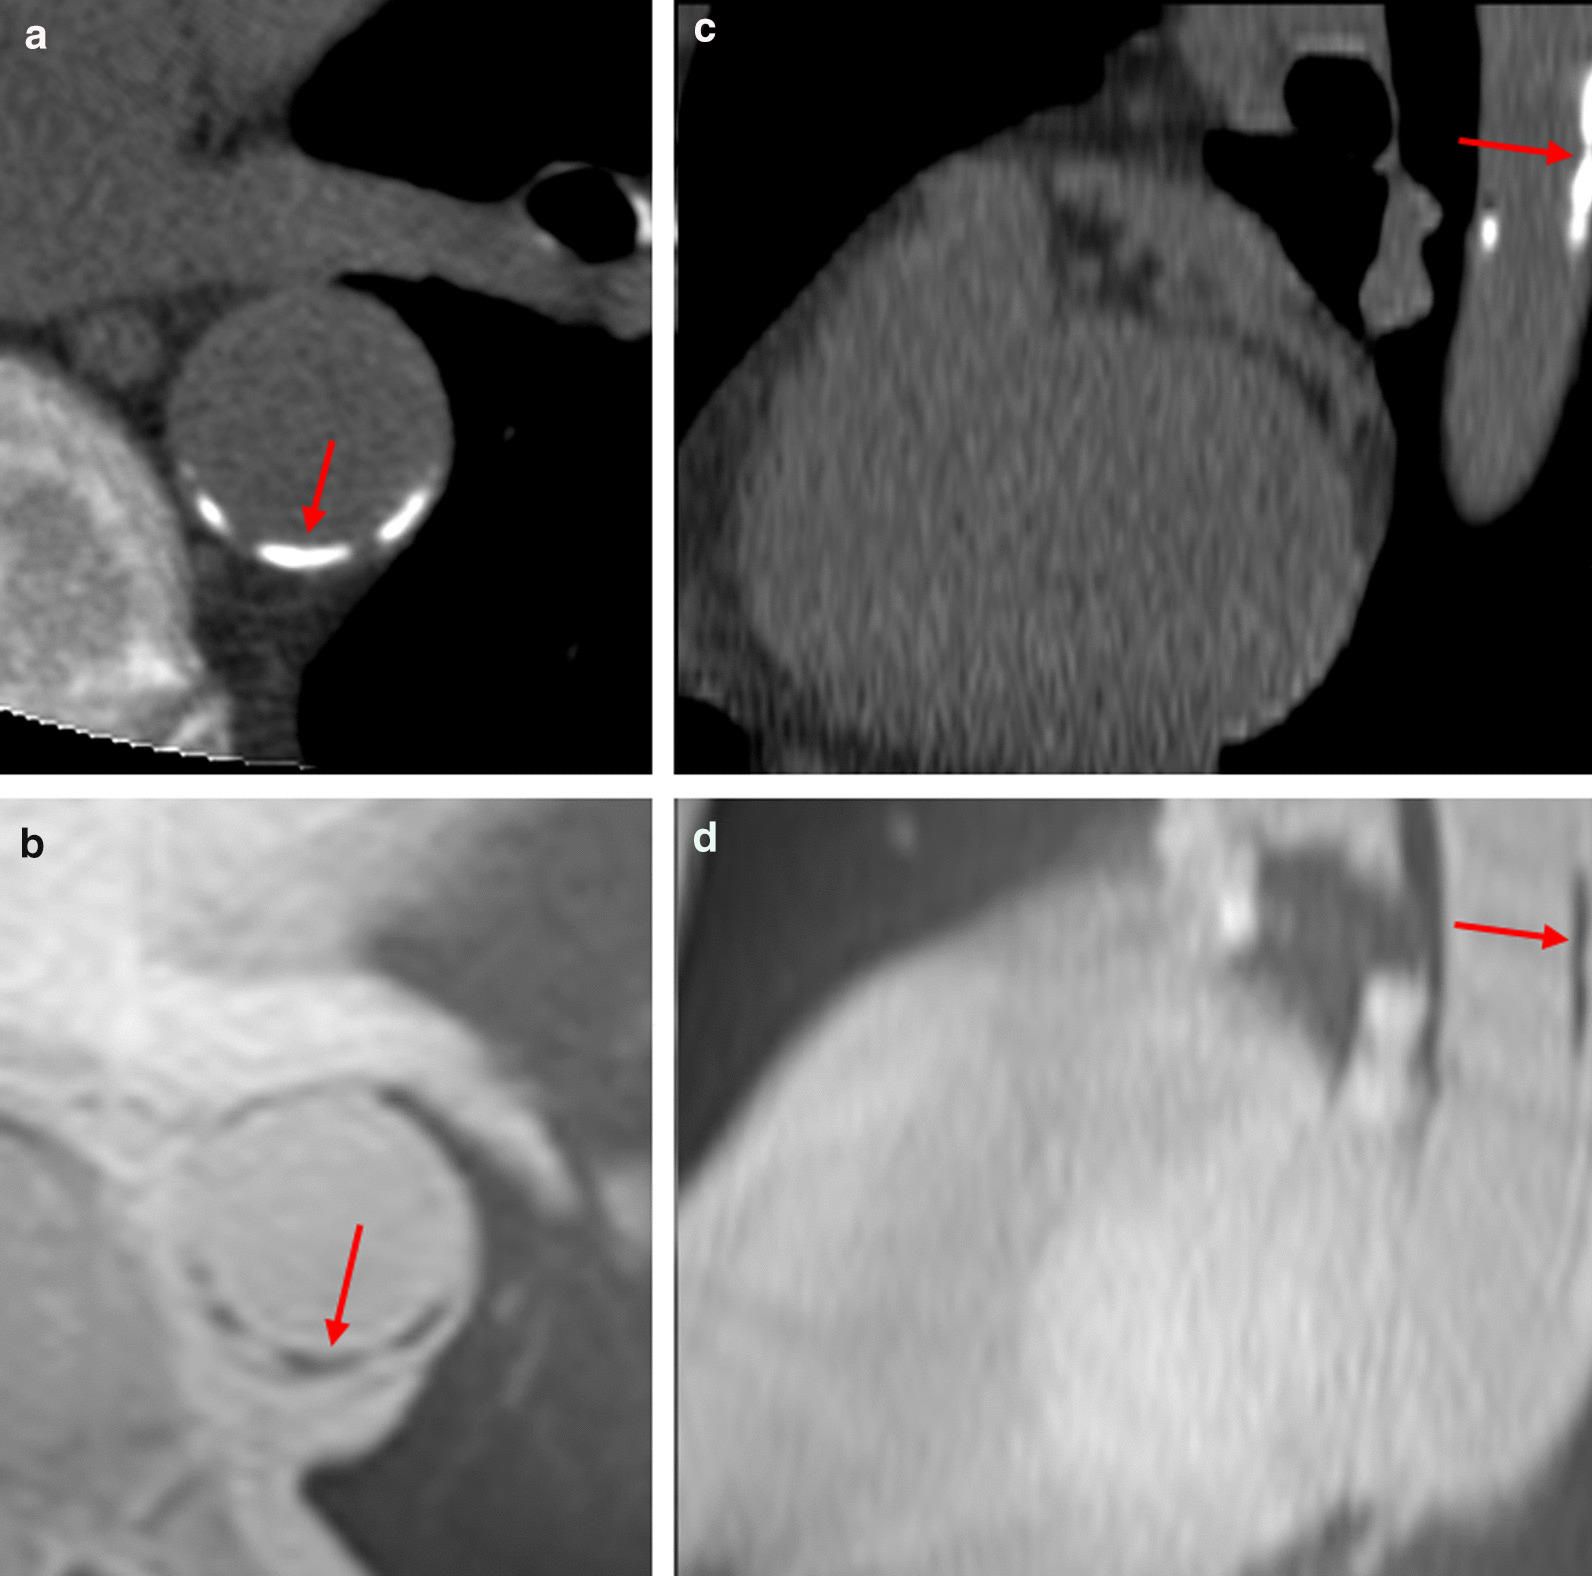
Representative images of calcifications on CT (a, c) and radial-VIBE (b, d). Images a and b are axial slices; Images c and d are sagittal slices. Calcifications are indicated by the red arrows. For this participant, volume of calcification detected by CT = 835 mm^3^, radial-VIBE volume = 634 mm^3^
